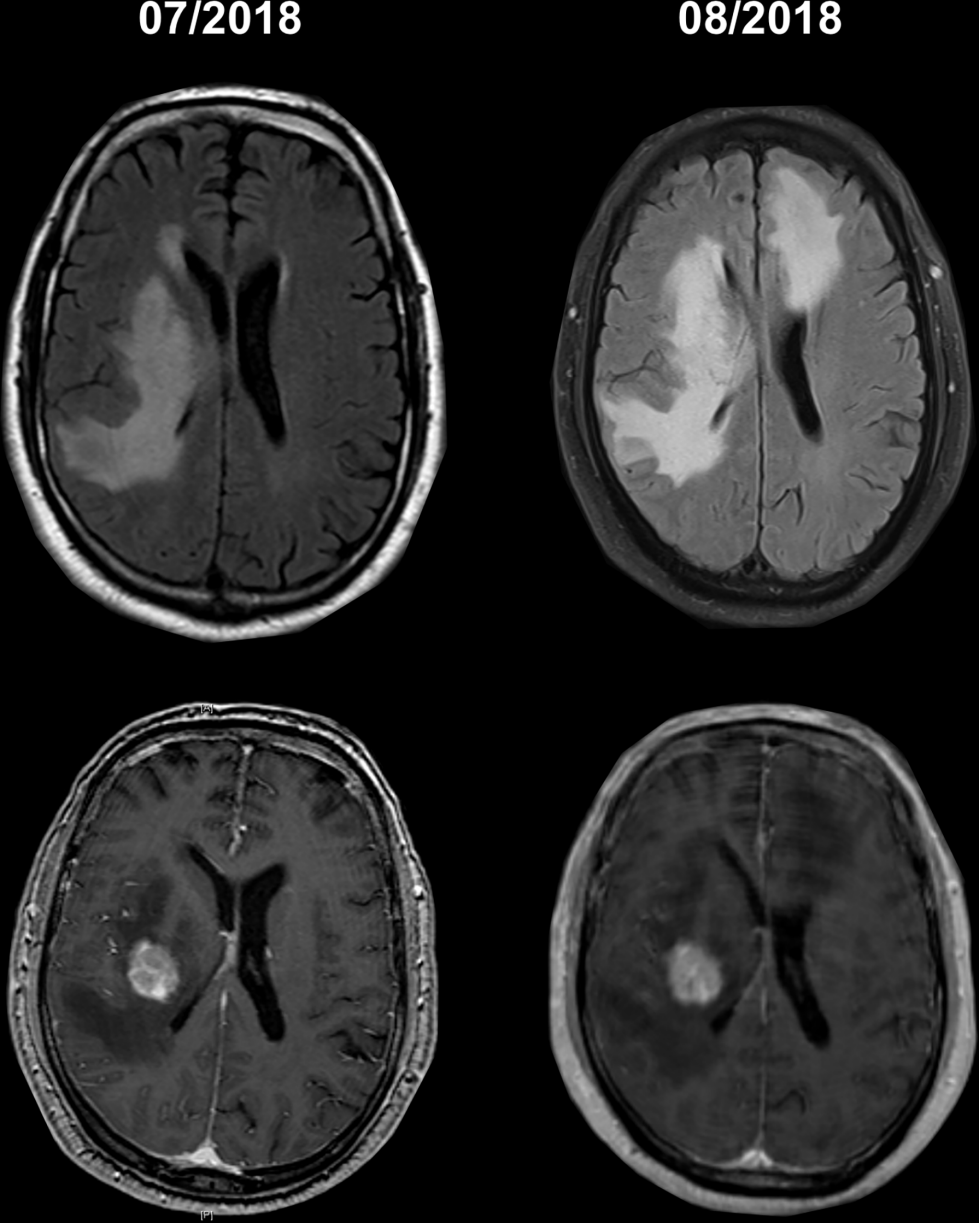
Cranial MRI scans of a 64-years-old with a metastasis malignant melanoma. The patient had been treated with radiosurgery of one metastasis (second row with contrast agent) and nivolumab after the radiation. After clinical deterioration within 4 weeks of starting checkpoint therapy, the patient showed a marked increase in T2 changes outside the radiation field.
